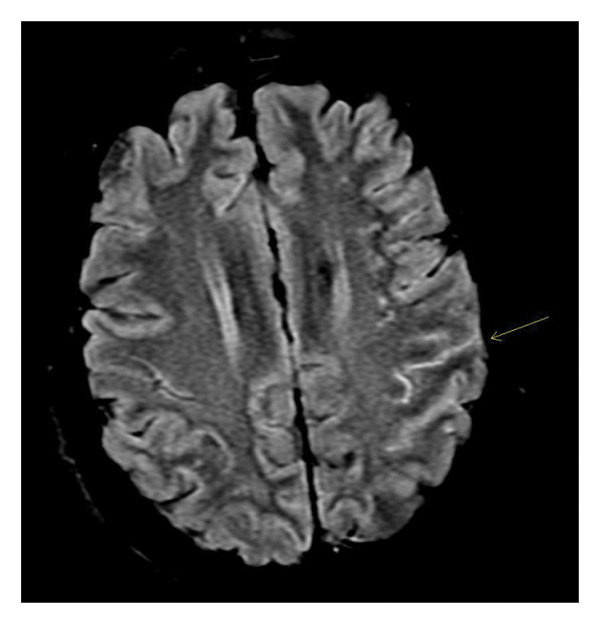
MRI brain, axial T2 FLAIR sequence (calvaria not visible as image brightness was adjusted to optimize visualization of leptomeningeal signal).
